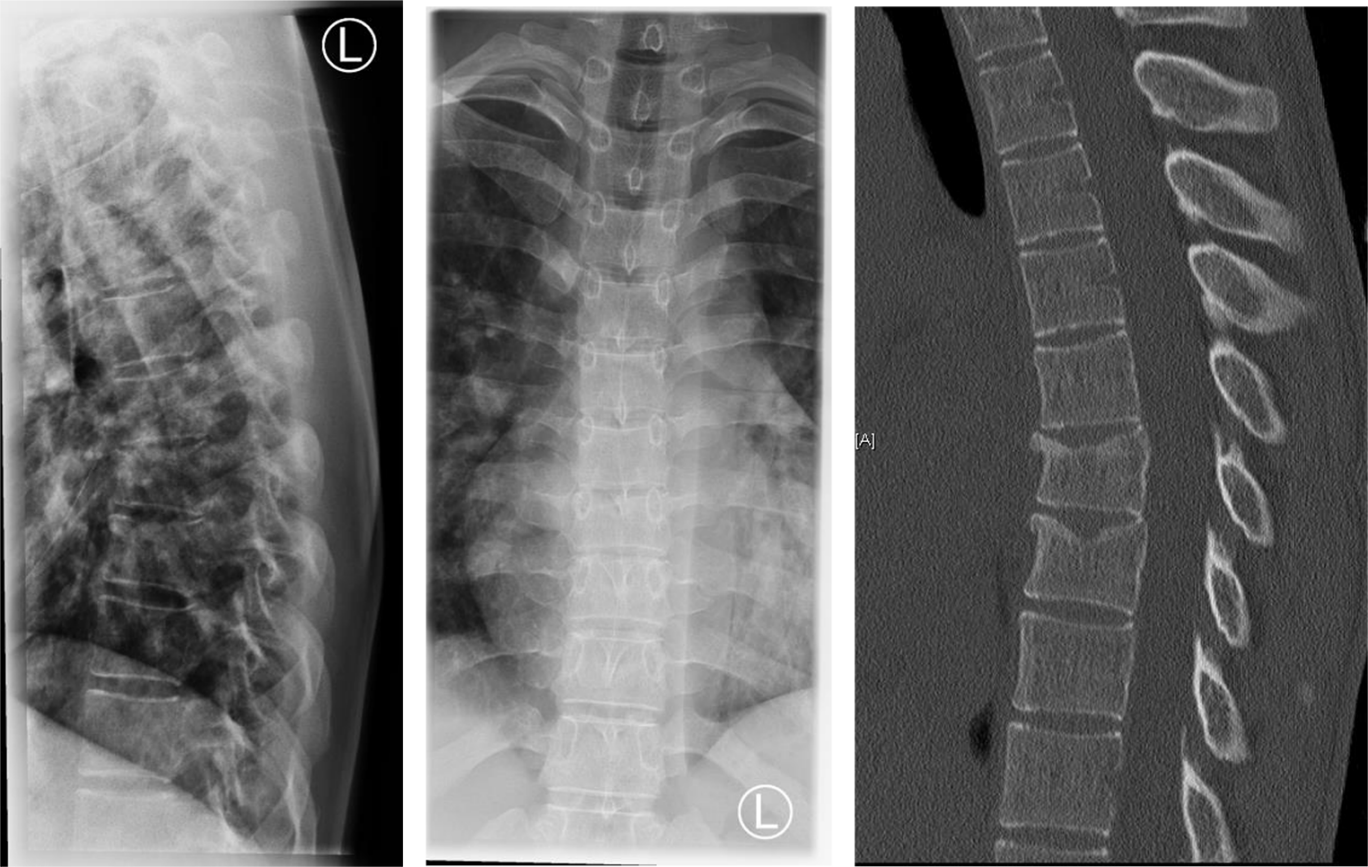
22 years, female, jump from 10 m diving board, AO type A3 fracture, Due to the age and moderate pain an MRI was not performed and conservative treatment was initiated
